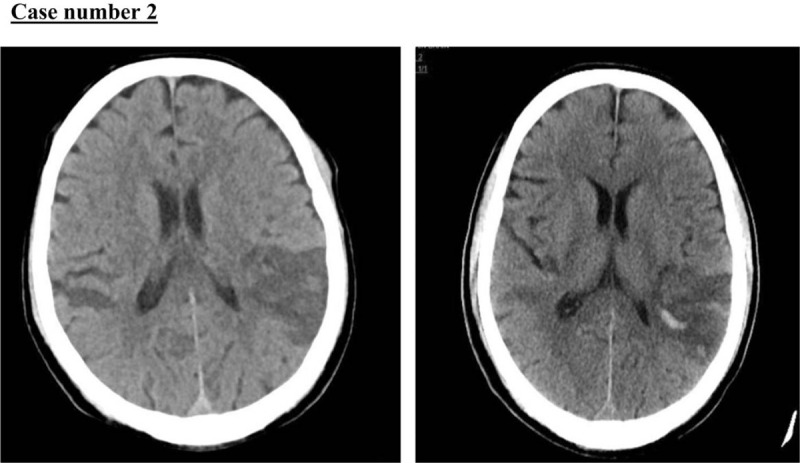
A and B unenhanced cranial computed tomography (CT) revealed multiple bilateral parietal, occipital, and cerebellar ischemic infarctions.
